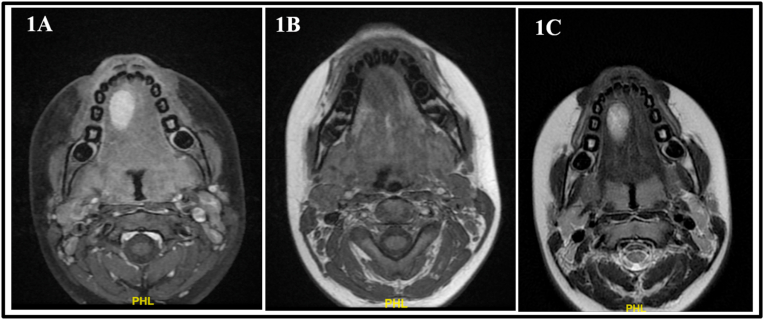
Includes 1A, 1B, 1C and shows the MRI findings demonstrating a well-defined lesion measuring 2.3 × 1.4 × 2.0 cm in size in AP, transverse and craniocaudal dimensions, respectively; note that the lesion is embedded within the intrinsic muscle of the oral tongue on the right side smoothly indenting the genioglossus/geniohyoid muscle complex, which is minimally displaced to the left side. Figure 1A: Axial T1-weighted image with contrast showing avid enhancement on post contrast images with no evidence of diffusion restriction. Fig. 1B: Axial T1-weighted image show the hypointense lesion.Fig. 1C: Axial T2-weighted image show heterogenous hyperintensity.
